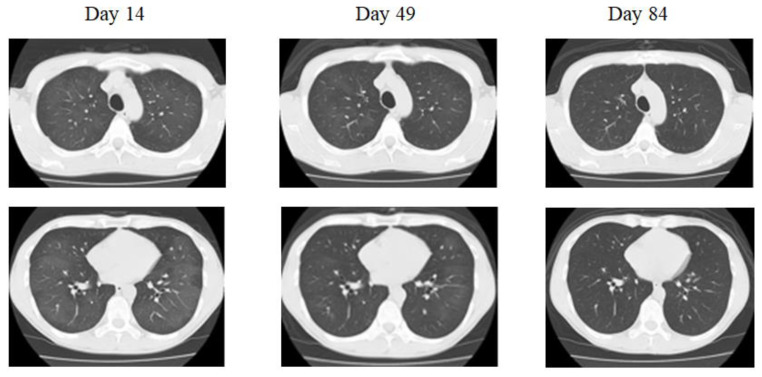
Chest X-ray and chest computed tomography (CT) in case 1. Bilateral diffuse, ground-glass opacity can be observed, but no pleural nadir shadow. A mosaic pattern can be observed in some areas (Day 14). There is a trend toward shadow reduction in both the upper and lower lung fields, but shadows are still present (Day 49). Improved completely (Day 84).
